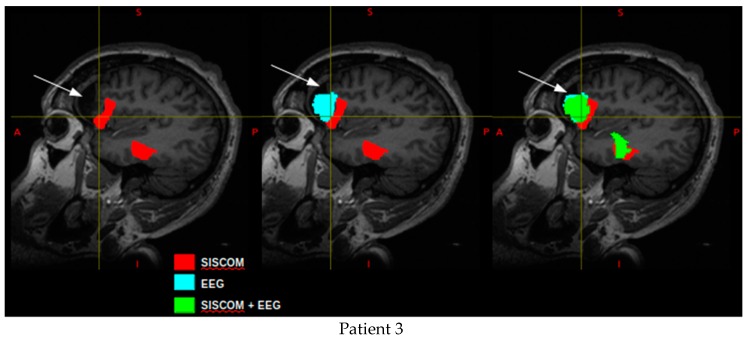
EZ estimated by the proposed methodology and co-registered to post-operative T1-weighted MRI: SISCOM (in red), ictal ESI (blue) and ictal ESI using SISCOM as a prior (green) for patient 3. The white arrow indicates the resection site.
